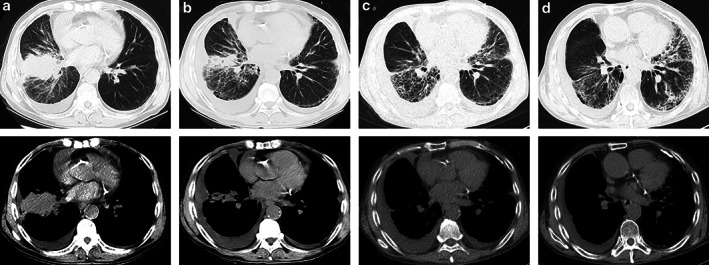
Chest computed tomography (CT) scan. (a) Before starting treatment, (b) After the first course of pemetrexed and carboplatin (AC) + pembrolizumab treatment. (c) After the second course of AC + pembrolizumab treatment; (d) After three weeks of steroid therapy
